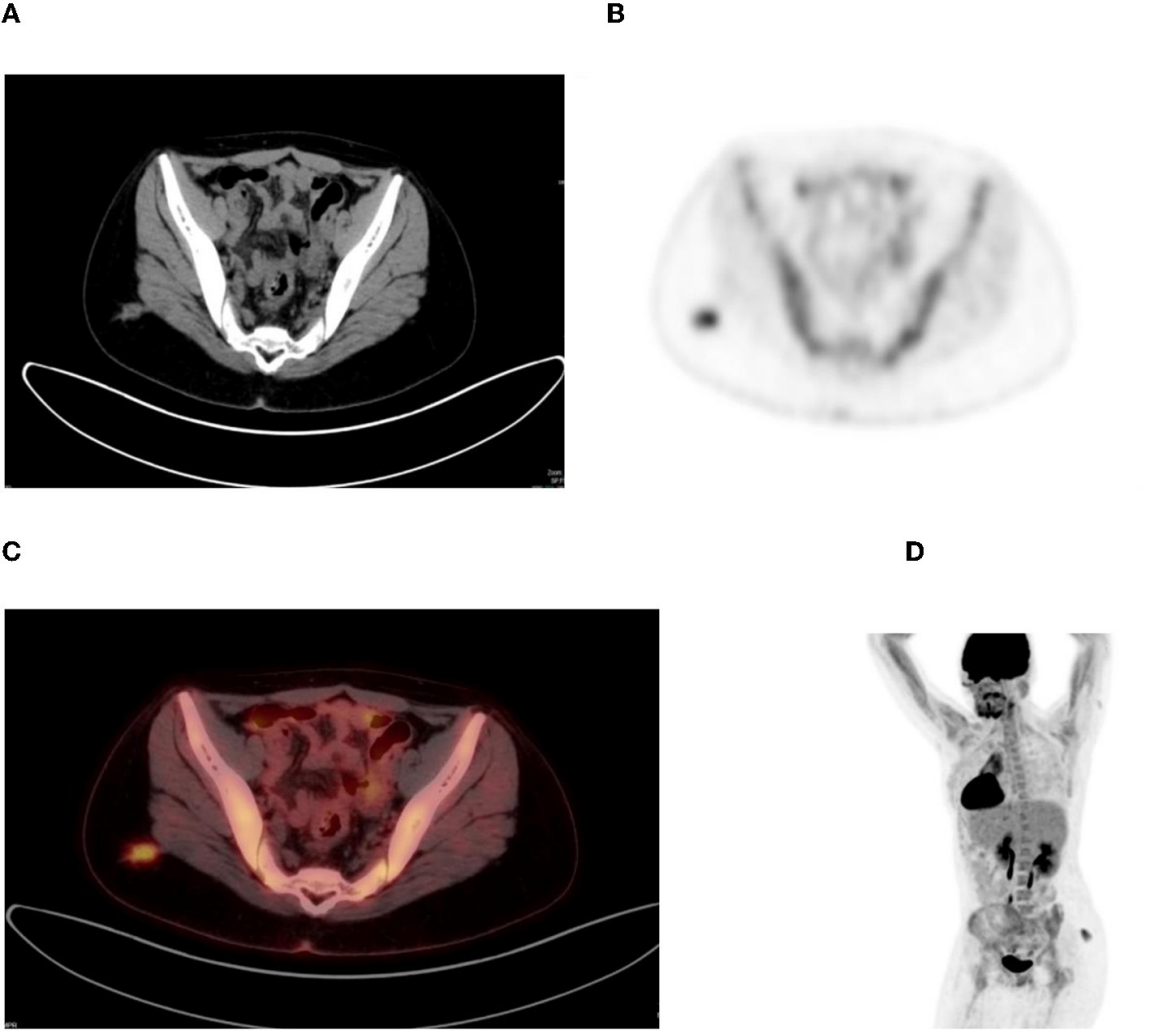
Young woman with Hodgkin's disease and underlying retroviral disease. CT (A), PET (B), combined PET/CT (C), and MIP (D) images show focal increased uptake in the right buttock, most likely inflammatory.
